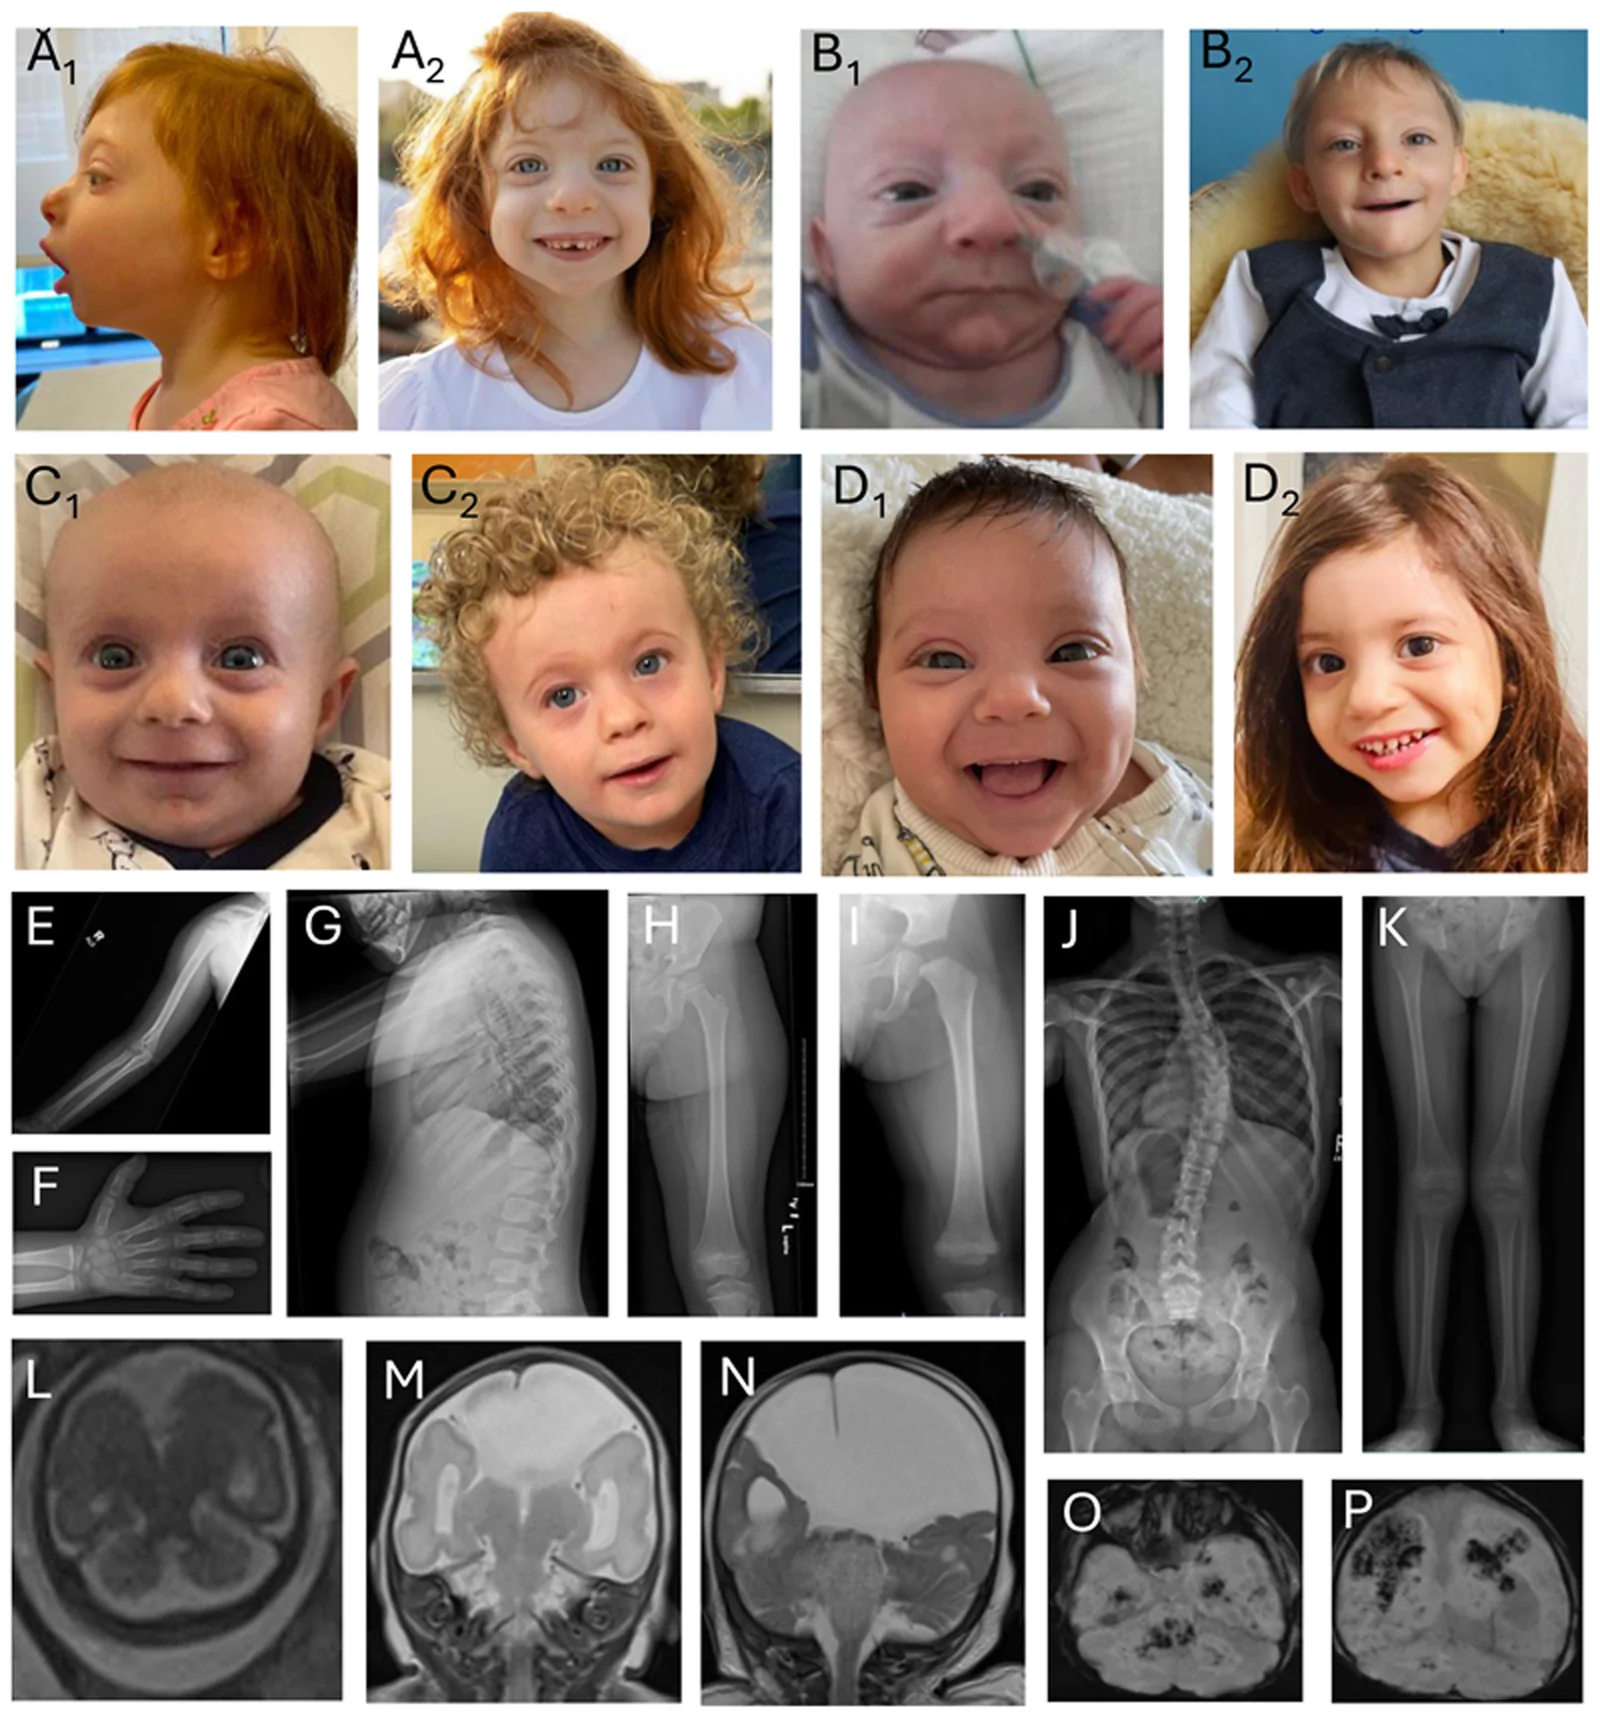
Photographs and imaging of individuals with RNU4ATAC-opathy. A-D. Facial features of four individuals with RNU4atac-opathy. Note the microcephaly, prominent eyes with epicanthus, infraorbital creases, slightly arched eyebrows, pointed chin, bulbous nasal tip, low set ears, and wide smile with slightly widely spaced teeth and thin upper vermilion of the lip. A_1_/A_2_. ID002 at 2 years and 3.5 years of age. Also note the mild retrognathia. B_1_/B_2_. ID016 at 3 months and 5.5 years of age. C_1_/C_2_. ID026 at 4 months and 2 years of age D_1_/D_2_. ID052 at 2 months and 4 years of age E-H. Radiographs of a 6-year-old female (ID001). Despite skeletal involvement in most individuals, no skeletal dysplasia was identified in the upper extremities (E), hands (F), spine (G), or lower extremities (H). I. AP lower extremity radiograph of a 2-year-old female (ID002), showing epiphyseal dysplasia identified after confirmation of the RNU4ATAC-opathy diagnosis. J. AP spine radiograph of a 17-year-old female (ID019), demonstrating thoracic scoliosis. K. AP lower extremity radiograph of a 6-year-old female (ID023), showing subtle mesomelia, epiphyseal dysplasia, and metaphyseal involvement L-P. MRI images of a male (ID013) at various ages. L. Fetal MRI at 28 weeks’ gestation showing lobar holoprosencephaly with significantly underdeveloped frontal, parietal, and occipital lobes M/N. Coronal T2 images at 2 weeks of age (N) showing a large dorsal intracranial cyst splaying the cerebral hemispheres, and at 12 months (O) showing cyst progression. O. Axial SWI at 12 months showing microhemorrhages in the brainstem, temporal lobes, and cerebellum. P. Coronal SWI at 12 months showing microhemorrhages in the temporal lobes, basal ganglia, and cerebellum.
